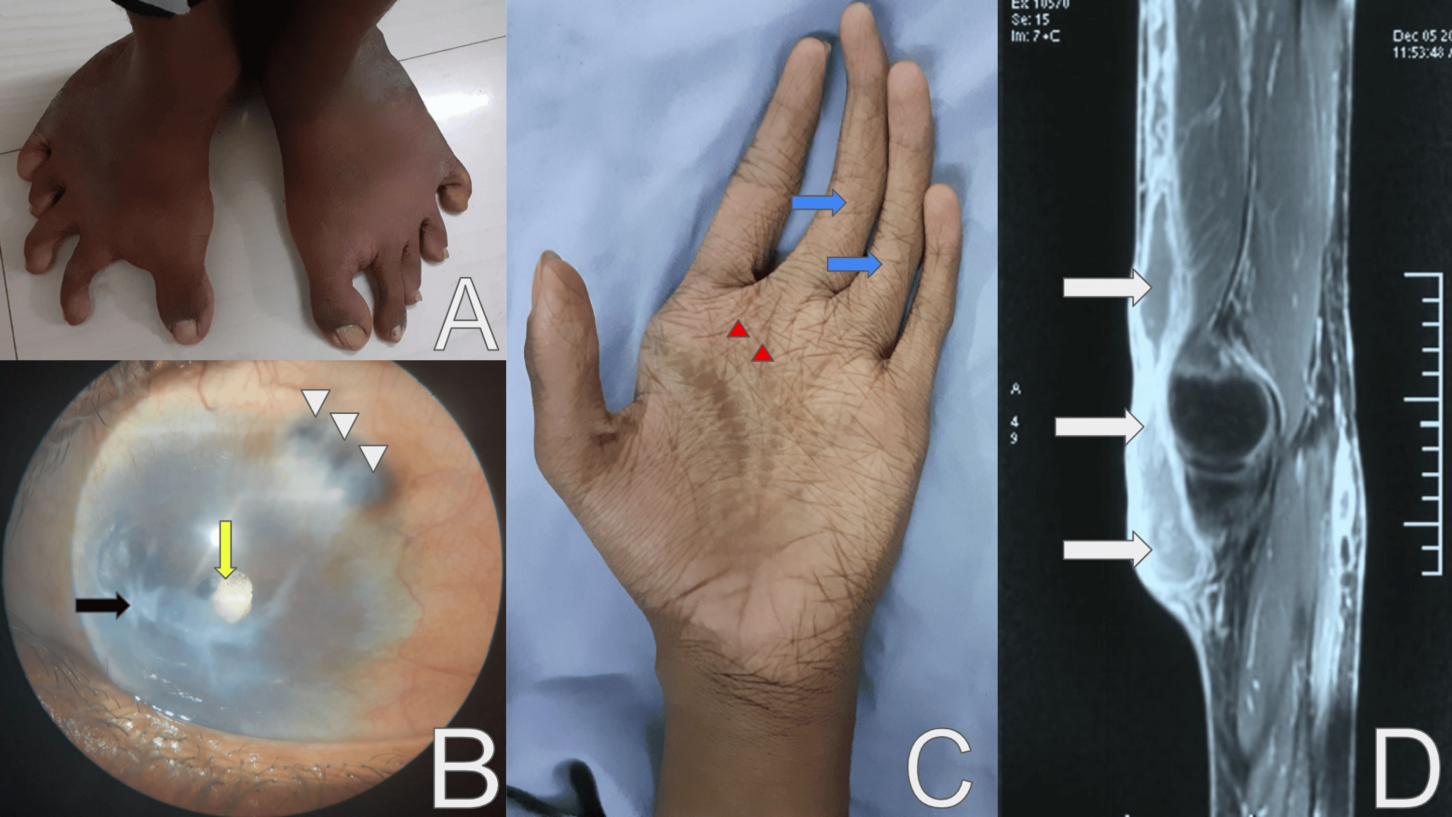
Case one findings A: bilateral congenital clubfeet; B: ciliary staphyloma in upper temporal quadrant (white triangles), corneal opacification (black solid arrow) and cataract (yellow arrow) in the left eye; C: spider-like long and slender digits (arachnodactyly; blue solid arrows) and increased palmar creases (red triangles); D: MRI of left knee showing hematoma extending from the anterior of mid-thigh to upper third of the leg (white solid arrows).
